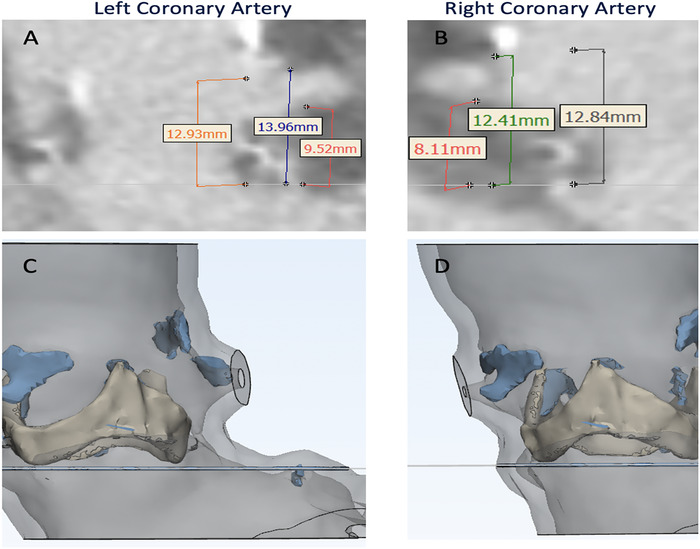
Computed tomography angiography evaluation of coronary ostial height shows (A) the left coronary artery inferior height of 9.52 mm, superior of 13.96 mm, and frame of 12.93 mm, while (B) the right coronary artery has an inferior height of 8.11 mm, superior of 12.41 mm, and frame of 12.84 mm. Views C and D show 3-dimensional reconstructions of both coronary ostia.
